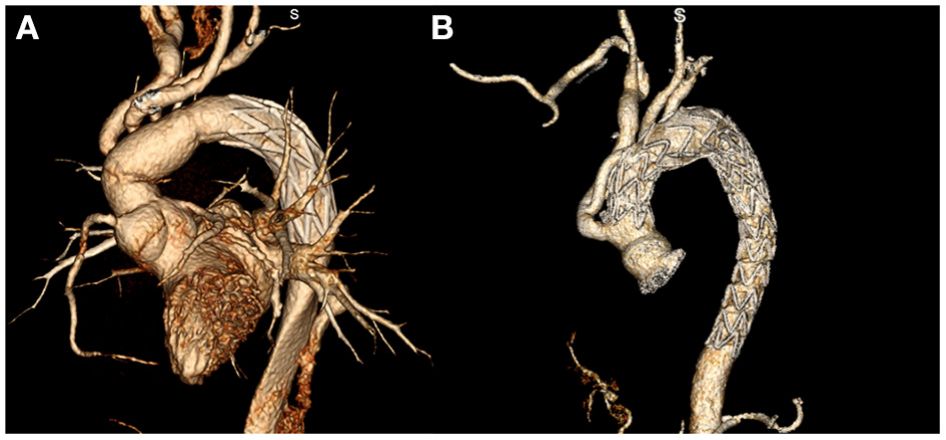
Post-operative aortic CTA reconstruction images of patients with acute aortic syndrome treated with total arch replacement combined with frozen elephant trunk implantation (A) and hybrid debranching surgery (B).
